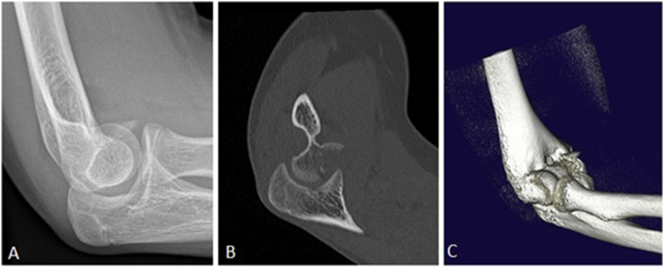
A: Lateral view of right elbow with suspicion of a coronal shear injury. B: Sagittal CT scan of coronal shear injury. C: 3D reconstruction of the joint.
